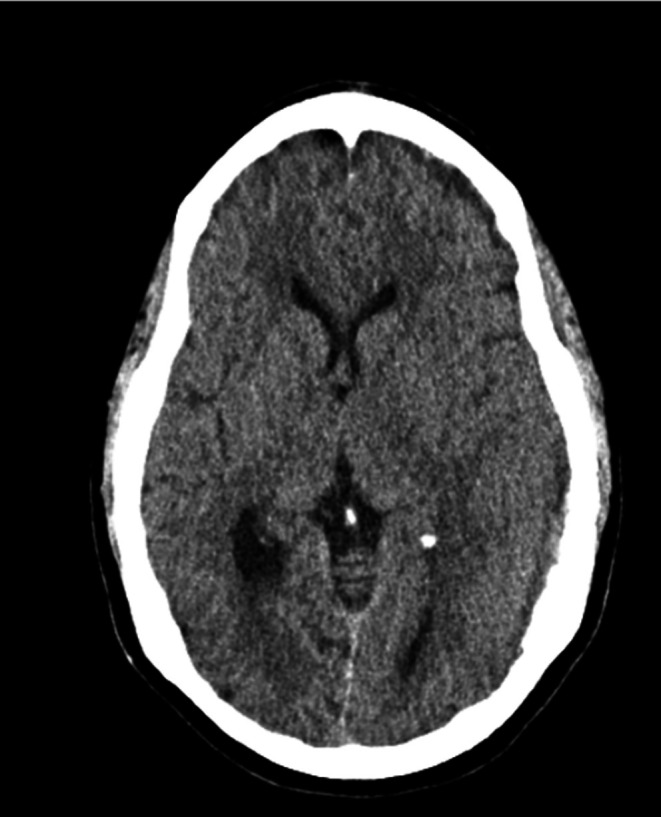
Post‐operative CT scan showing no significant change in the mostly chronic left and mostly posterior convexity subdural hematoma measuring up to 11 mm on re‐admission following fall at POD 18.
